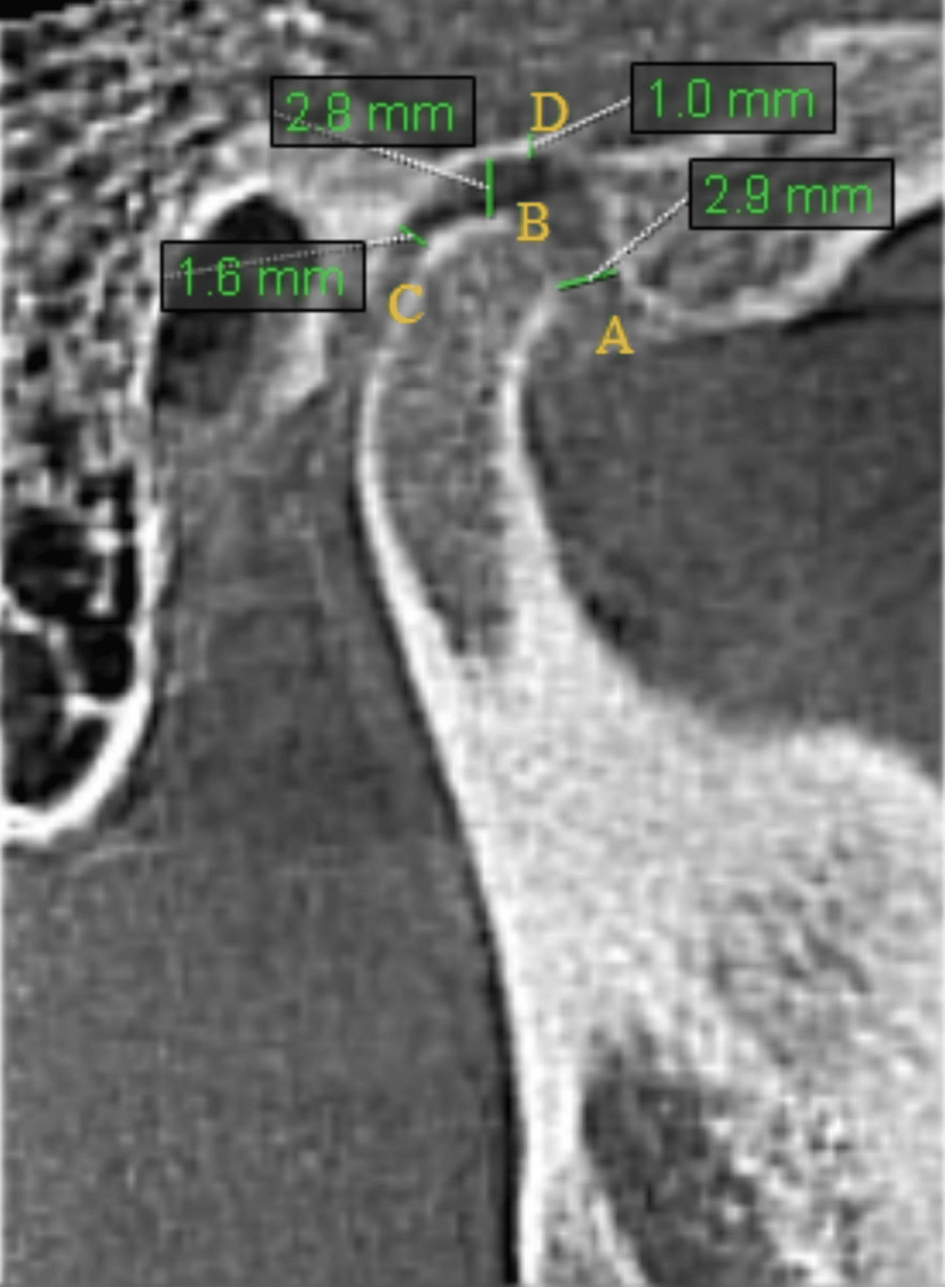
Reconstructed sagittal slice of cone beam computed tomography (CBCT) image. (A) Anterior joint space, (B) superior joint space, (C) posterior joint space, (D) glenoid fossa roof thickness
